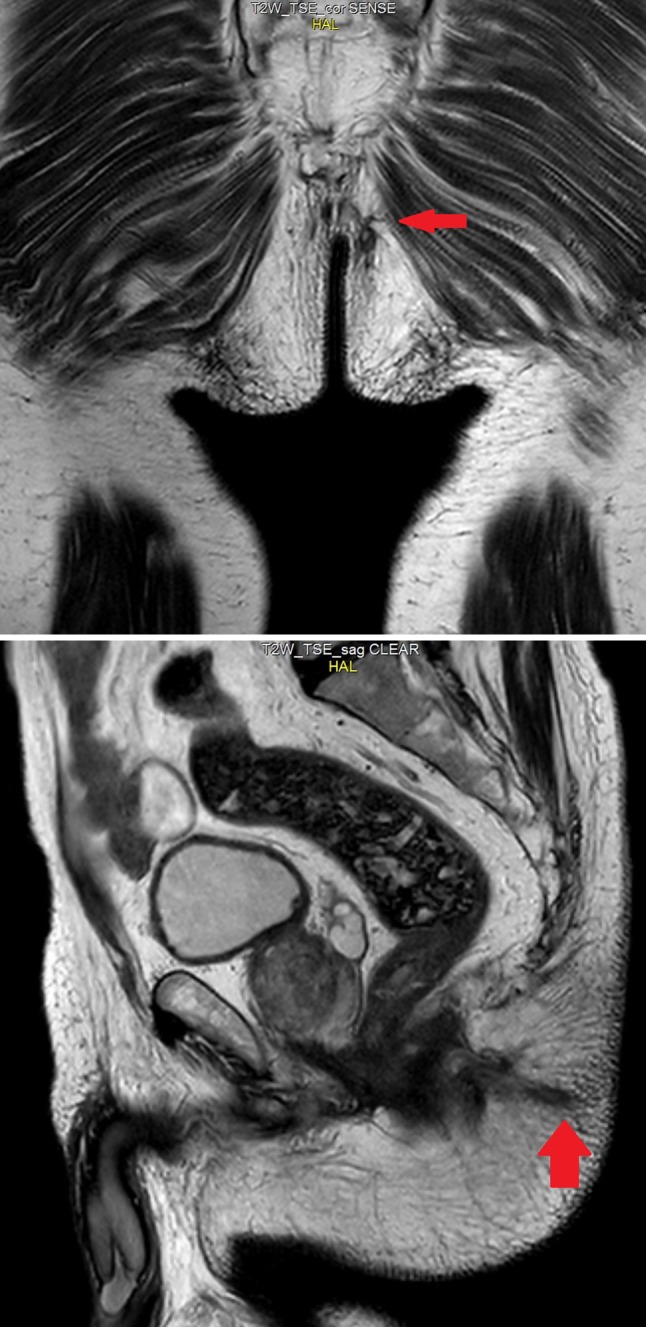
Pelvic magnetic resonance imaging with evidence of transphinteric fistula (red arrow) of the high-mid anal canal without any internal opening and another fistula extending as far as the left internal obturator muscle
